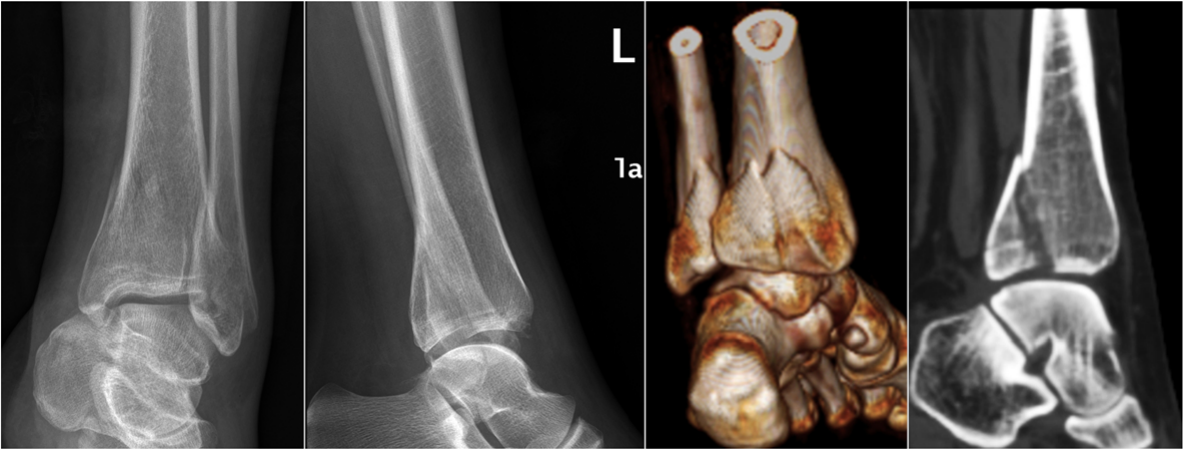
The 56-year-old female patient treated using direct reduction technique. Preoperative AP and lateral radiograph showed a pilon-type PMF. Preoperative 3D and sagittal reconstruction of CT images provided more precise determination of the morphology and size of the posterior fragments
